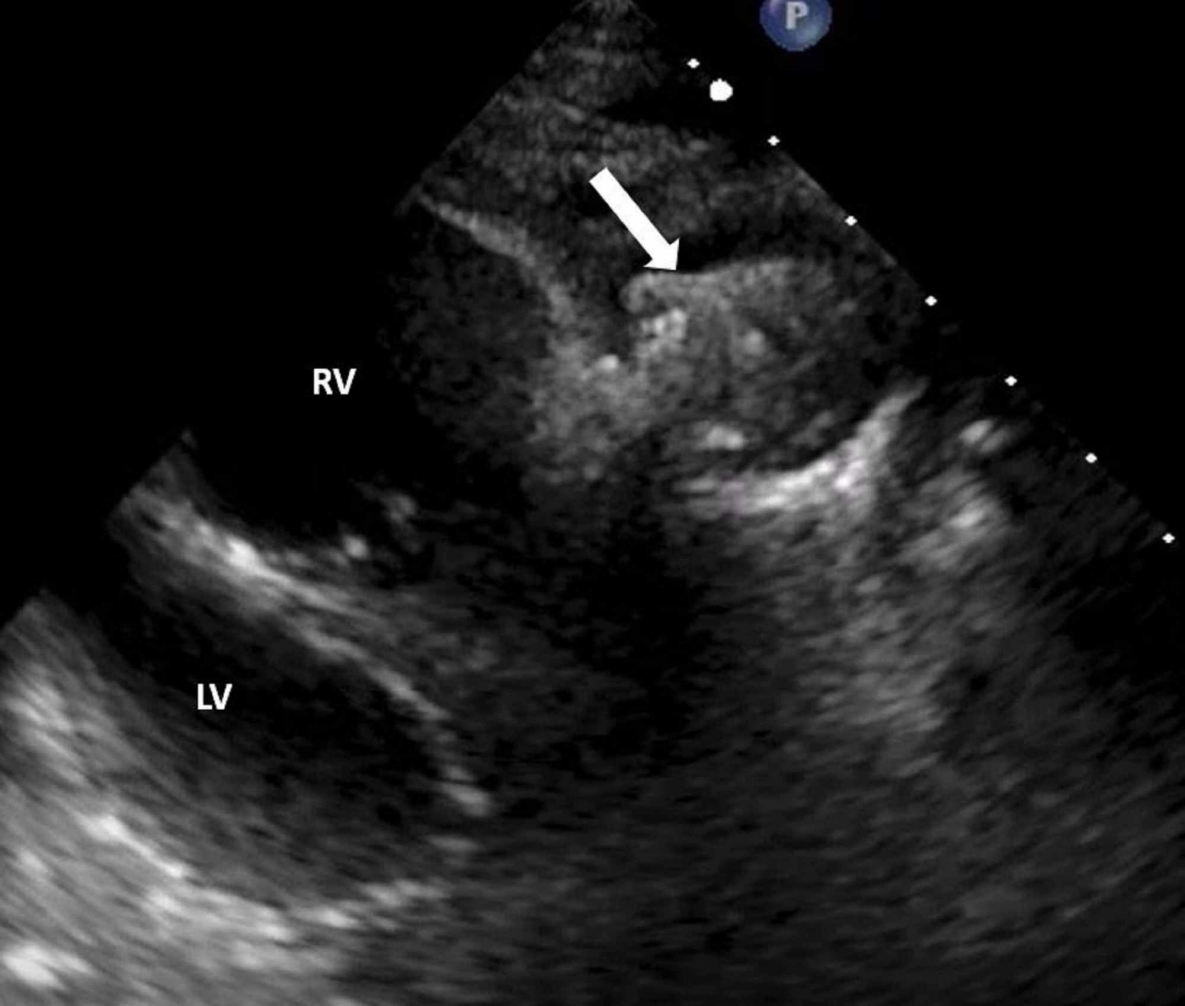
TTE in a 17-year-old patient with transcatheter pulmonary valve and features suspicious of infective endocarditis A still image from TTE shows suboptimal evaluation of the right ventricular outflow tract due to artifact from the transcatheter pulmonary valve (Melody valve) stent apparatus (arrow). RV, Right ventricle; LV, Left ventricle; TTE, Transthoracic echocardiogram
